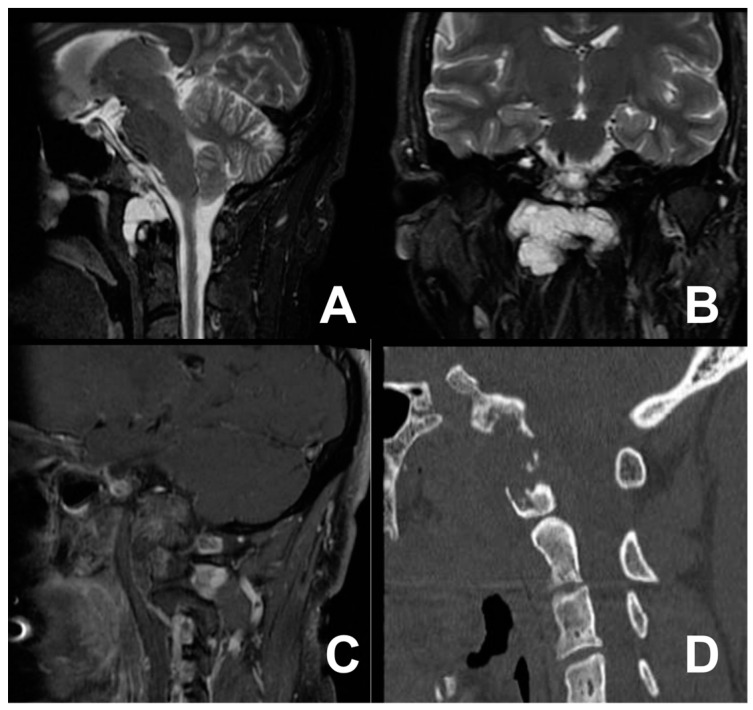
T2-weighted MRI scan in the sagittal (A) and coronal views (B) and T1 sagittal post-contrast image (C) showing the cervical tumor anteriorly extending toward the occipital condyles, involving the right anterior arch of the atlas, with spongy bone replacement and consequential destruction of the cortical and right side of the clivus (D).
